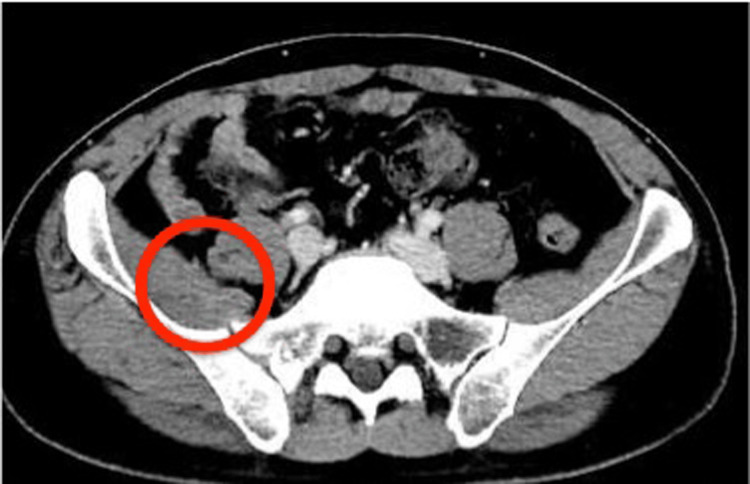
Follow-up contrast computed tomography scan on the 85th day after the admission date showing no abscess in the iliopsoas muscle.
